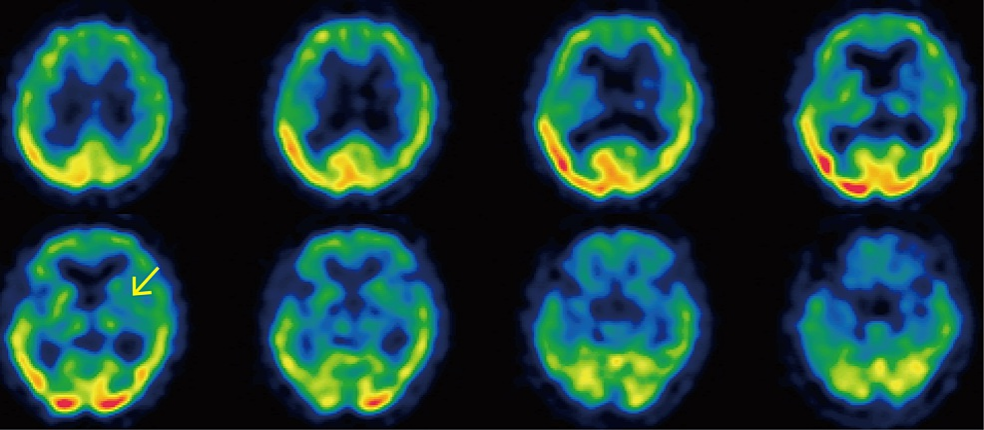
SPECT images SPECT showed hypoperfusion in the left putamen (arrow). The number of days was counted from the date of first admission. SPECT - single-photon emission computed tomography
